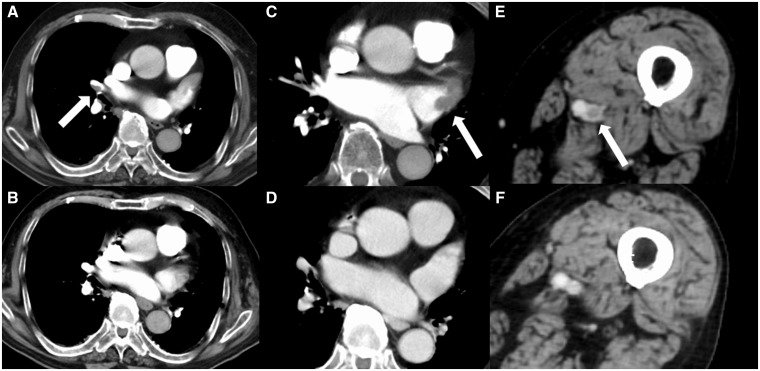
Time course of the pulmonary embolism (*A* and *B*), left atrial appendage thrombus (*C* and *D*), and deep venous thrombosis (*E* and *F*) on contrast-enhanced computed tomography. (*A*) A thrombus was detected in the right pulmonary artery (arrow). (*B*) This thrombus had completely disappeared by 1 month after discharge. (*C*) A thrombus was detected in the left atrial appendage (arrow). (*D*) No thrombi were detected at 1 month after discharge. (*E*) A thrombus was detected in the left superficial femoral vein (arrow). (*F*) No thrombi were detected at 1 month after discharge.
